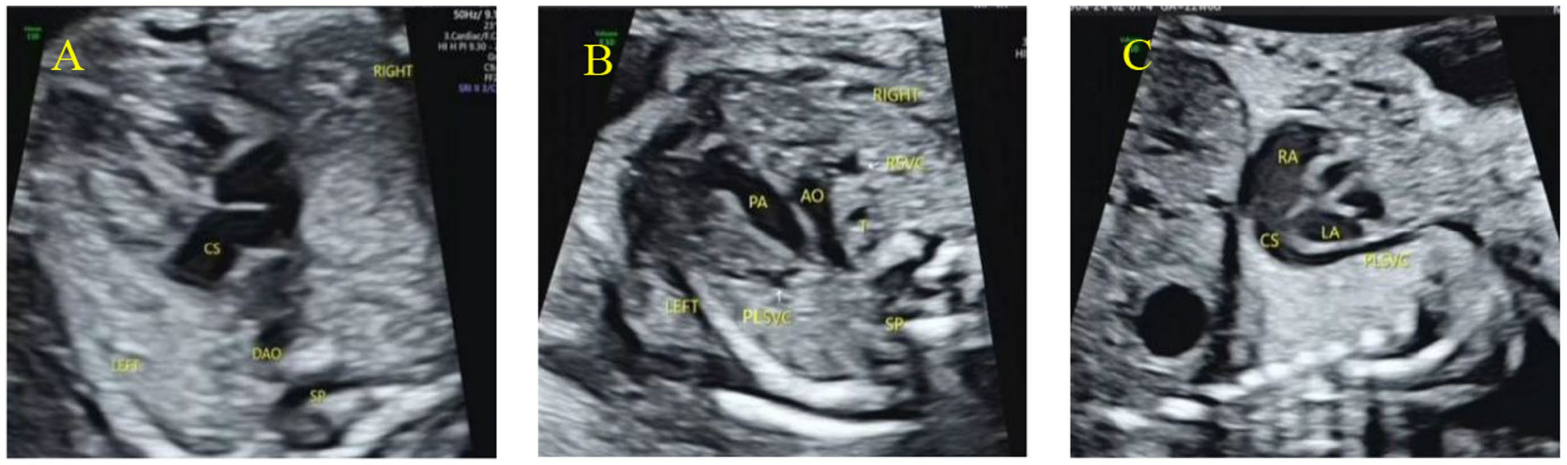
Primary ultrasound sections of fetal PLSVC. (A) The coronary sinus section shows the coronary sinus is widened; (B) the 3VT section shows the “four-vessel sign,” that is, the left superior vena cava appears on the left side of the pulmonary artery; (C) the paravertebral sagittal section of the neck and chest shows the left superior vena cava connecting to the dilated coronary sinus and draining into the right atrium.
